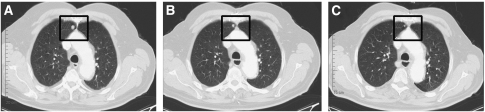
CT scans of an index lung metastasis from patient C. (A) Lung metastasis (square) at baseline just before initiating sunitinib. (B) Lung metastasis after 3 months sunitinib. (C) Lung metastasis after 7 months sunitinib.
